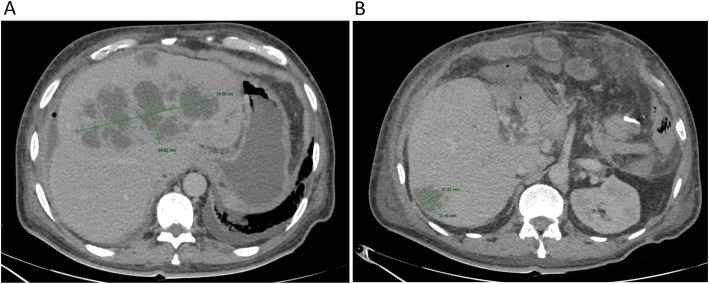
Computed Tomography (CT) scan showing an enlarged liver with (A) multiple heterogeneous hypodense fluid-filled lesions compatible with liver abscesses on both hepatic lobes. The largest abscess was localized on the liver segment VIII measured 14.5 cm × 5.4 cm. Another small abscess was present on liver segment IV. (B) A smaller abscess (3.1 cm × 2.7 cm) was present on the liver segment VI. Note the surgical absence of the gallbladder and the spleen
